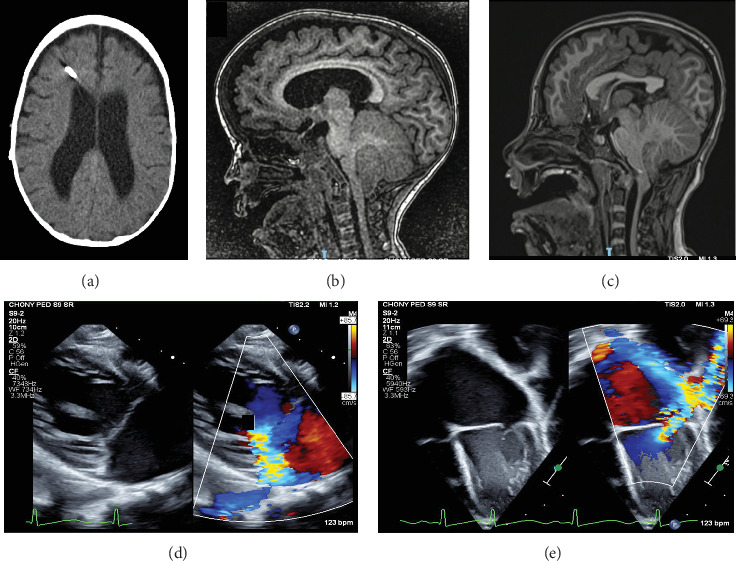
Imaging studies. (a) Case 1, axial CT imaging from 14 months of age showing ventricle dilation status postventriculoperitoneal shunt placement. (b) Case 1, Chiari I malformation with hydrocephalus, with cerebellar herniation and crowding of the foramen magnum at 1 year of age. (c) Case 2, Chiari II malformation with caudal displacement of the cerebellar tonsils, vermis and brainstem, elongation of the medulla, dysgenesis of the corpus callosum, and polymicrogyria at 8 years of age. (d, e) Case 1, preoperative echocardiogram images revealing severe mitral regurgitation in the long axis view (d) and apical view (e).
